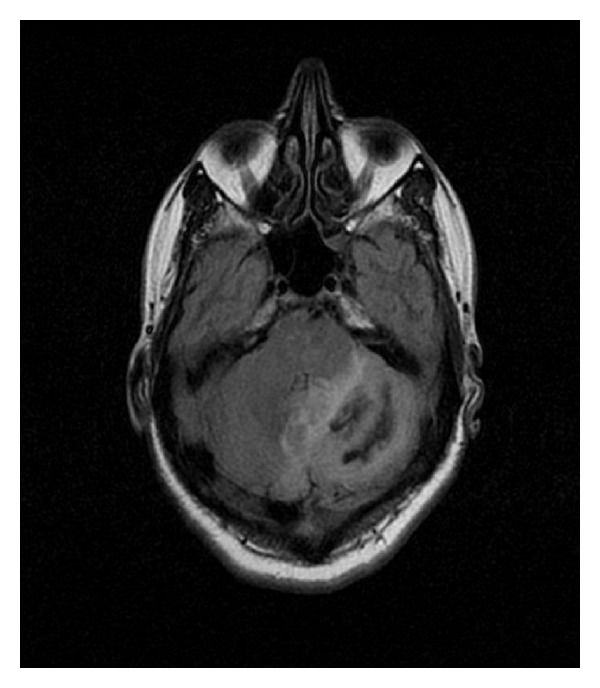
Cranial FLAIR enhancement MRI image showing left cerebellar hemorrhage.
